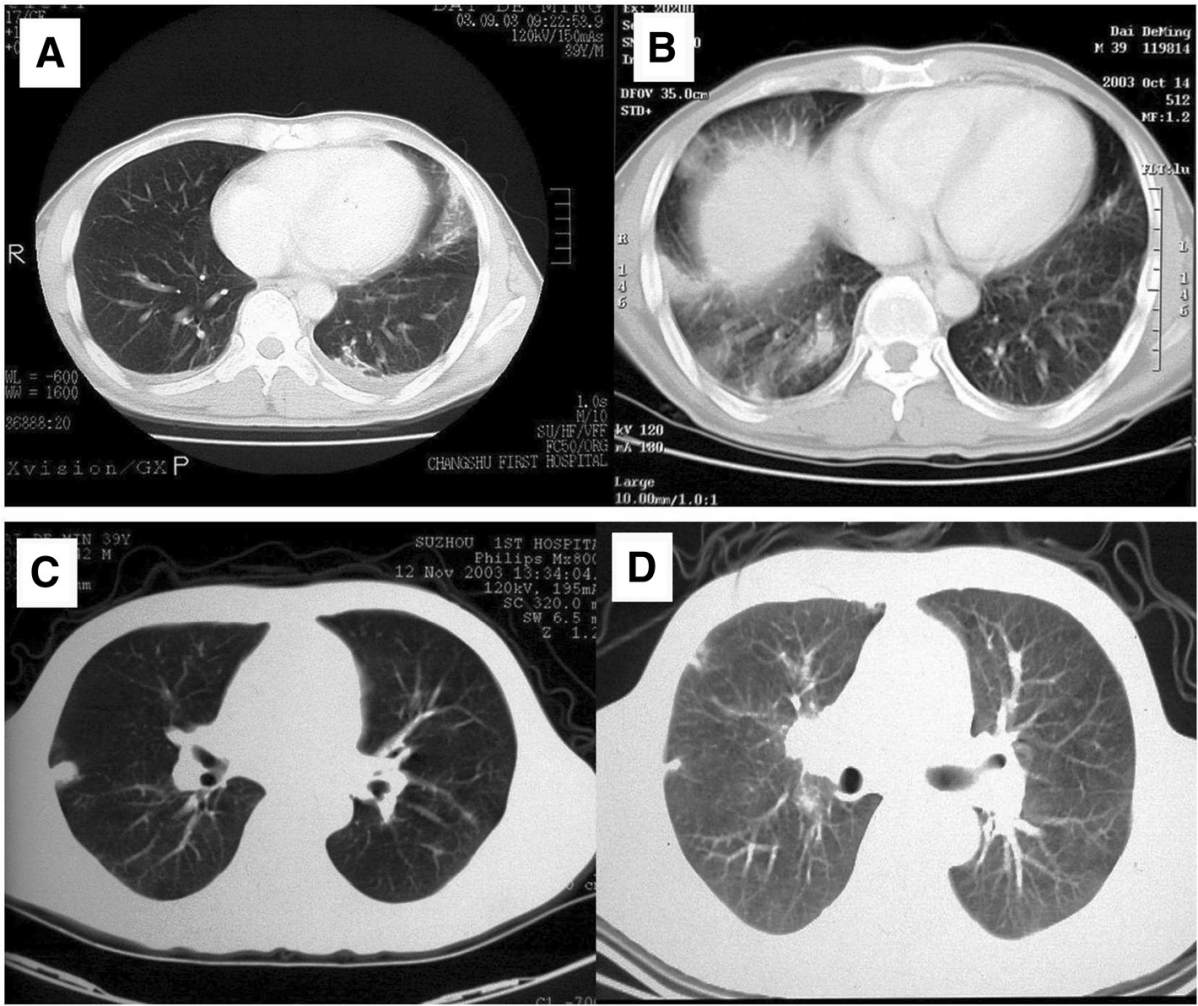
CT findings upon admission.
